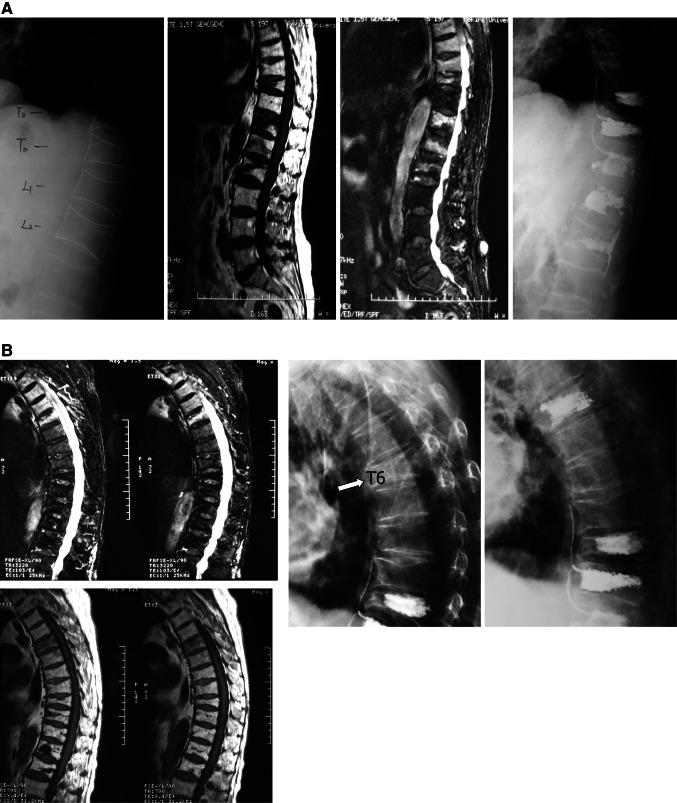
a Sagittal T1, T2-weighted MR and plain images show T10, T12–L2 OVCF of a 73-year-old man treated by PKP in February, 2008. T11 was treated prophylactically. b Ten months later, a new compression fracture at T6 was subjected to repeated PKP
